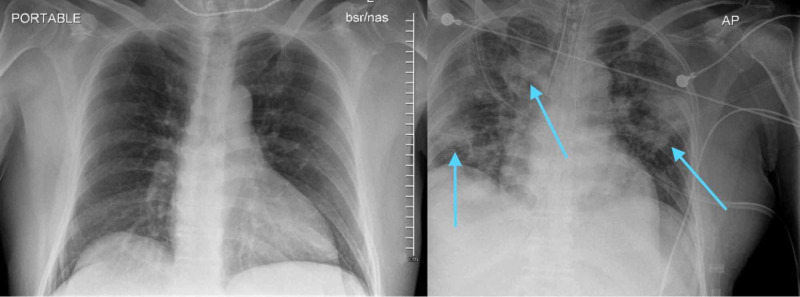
Comparison of chest X-ray changes on day 1 (left) and day 5 (right) of presentation.
